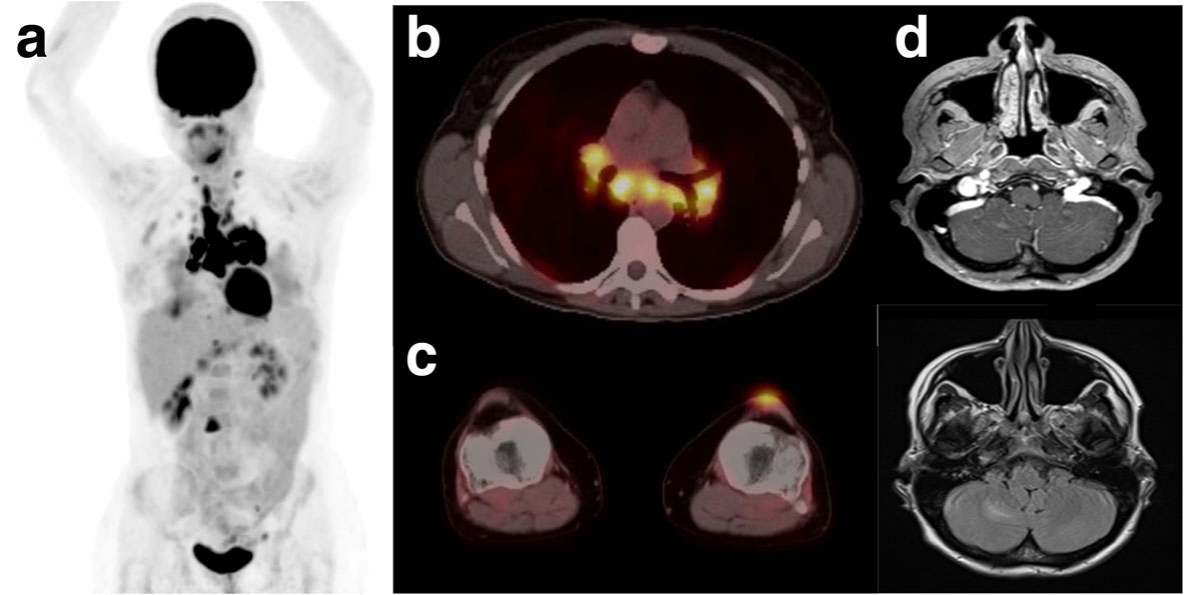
PET-CT scan after Nivolumab + Ipilimumab Induction and on Maintenance Nivolumab. a Scout PET scan showing cervical/mediastinal/inguinal adenopathy and vertebral lesions. b Cross section of mediastinum with increased uptake in mediastinal nodes (SUV_max_ 16 in paratracheal node). c Cross section of knees with increased uptake in left soft tissue nodule (SUV_max_ 2.7). d MRI brain with interval improvement of right cerebellar metastases
